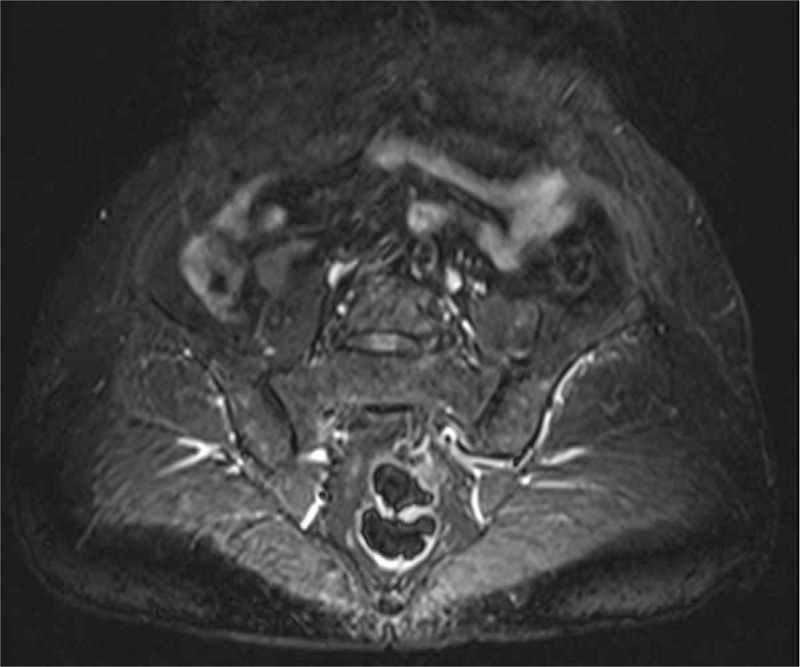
No abnormality was found in the MRI of the sacroiliac joint or the hips.
